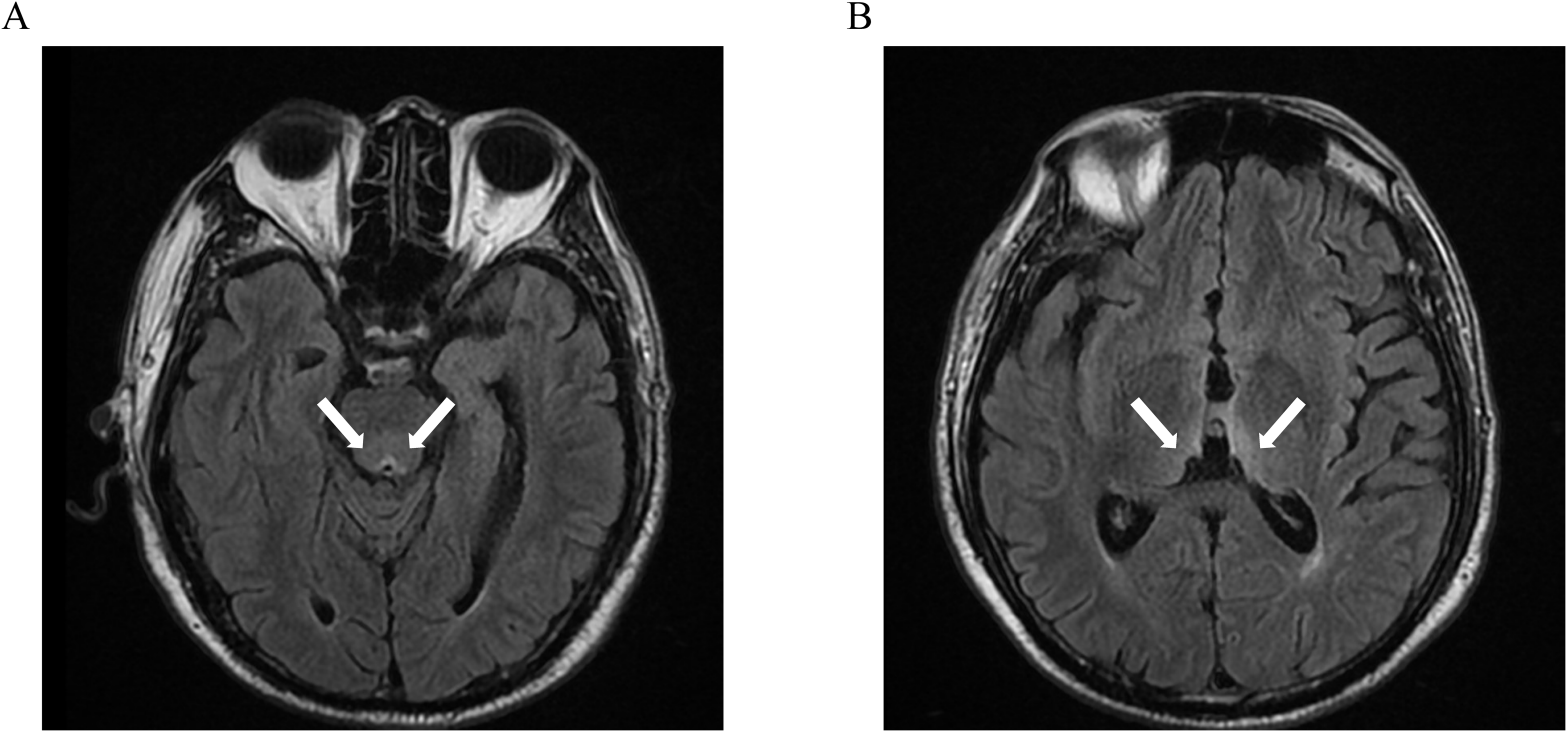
Symmetric T2-FLAIR hyperintense lesions involving the periaqueductal region (A), bilateral thalami, and the margins of the third ventricle (B).
